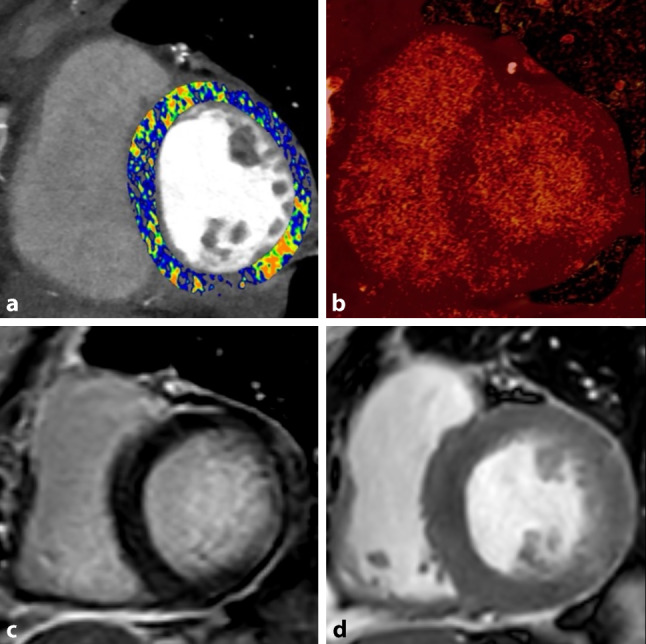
Short-axis photon-counting detector CT (PCD-CT, a and b) and cardiovascular magnetic resonance images (c and d) of a 59-year-old female recently diagnosed with myocardial infarction. *Image *a shows a PCD-CT extracellular volume (ECV) map of the left ventricle with focal increased ECV in the basal inferolateral segment. *Image *b shows a calculated iodine map with retainment of iodine in an ischaemic pattern, corresponding with an area of late gadolinium enhancement on cardiovascular magnetic resonance (*Image* c). *Image* d shows a short-axis cine image (after gadolinium administration) in which increased signal intensity and regional wall motion abnormalities were observed in the basal inferolateral segment, indicative of myocardial infarction
